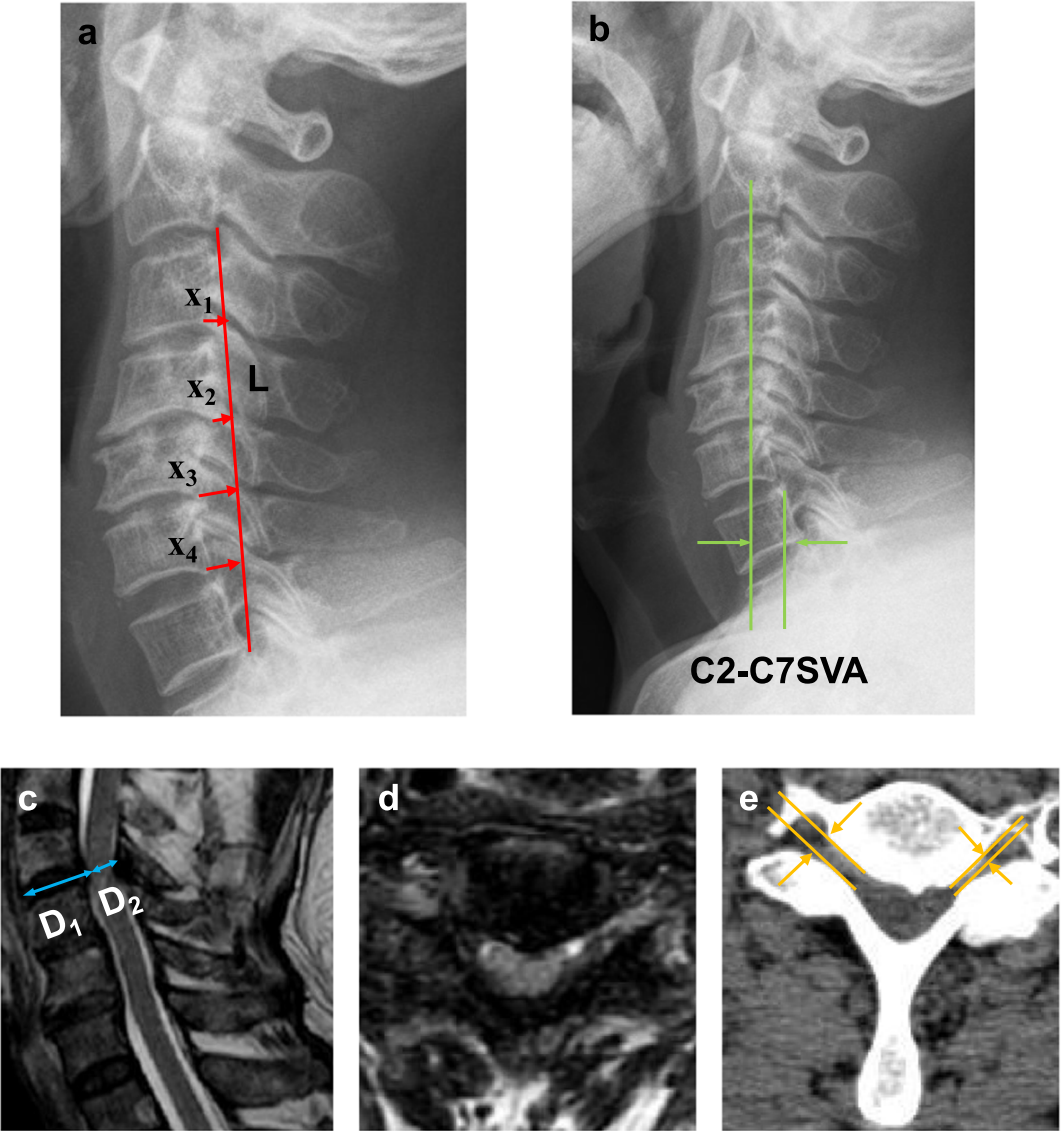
Radiographic evaluation. a CCI on cervical spine X-rays. CCI: Line x is the distance between the posterior inferior points of C2 and C7. The distances between the posterior inferior points of C3C6 and line L are called a1 to a4, respectively. The CCI is computed by the following formula: CCI = (*x*_1_ + *x*_2_ + *x*_3_ + *x*_4_)/L 100%. When posterior inferior points of C3C6 are behind line L, the values of *x*_1_ to *x*_4_ are negative. b C2-7 SVA on cervical spine X-rays. The distance between the center point through C2 and the vertical line of the posterosuperior corner of C7 was defined as C2C7 SVA. c Modified Pavlov ratio on sagittal T2-weighted MRI. Pavlov ratio (= *D*_1_/*D*_2_, vertebral body spinal canal ratio). d Presence of protruding lesions at the anterior portion of the spinal cord. e Foraminal stenosis between C4 and C5 on axial CT image. The yellow arrows indicate foraminal dimension
